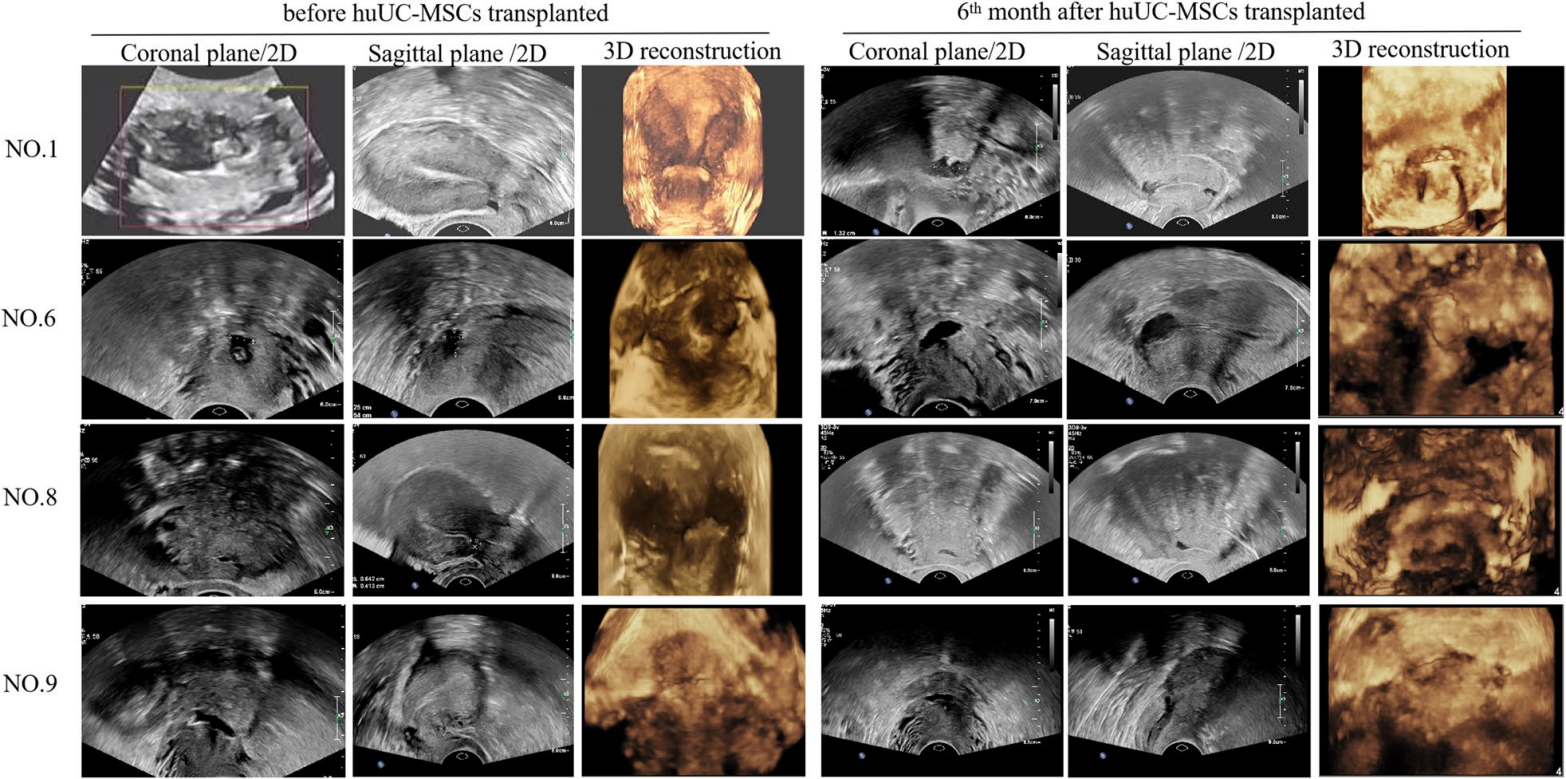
Doppler ultrasound imaging from 4 patients with cesarean scar diverticulum before and sixth month after human umbilical cord MSCs
